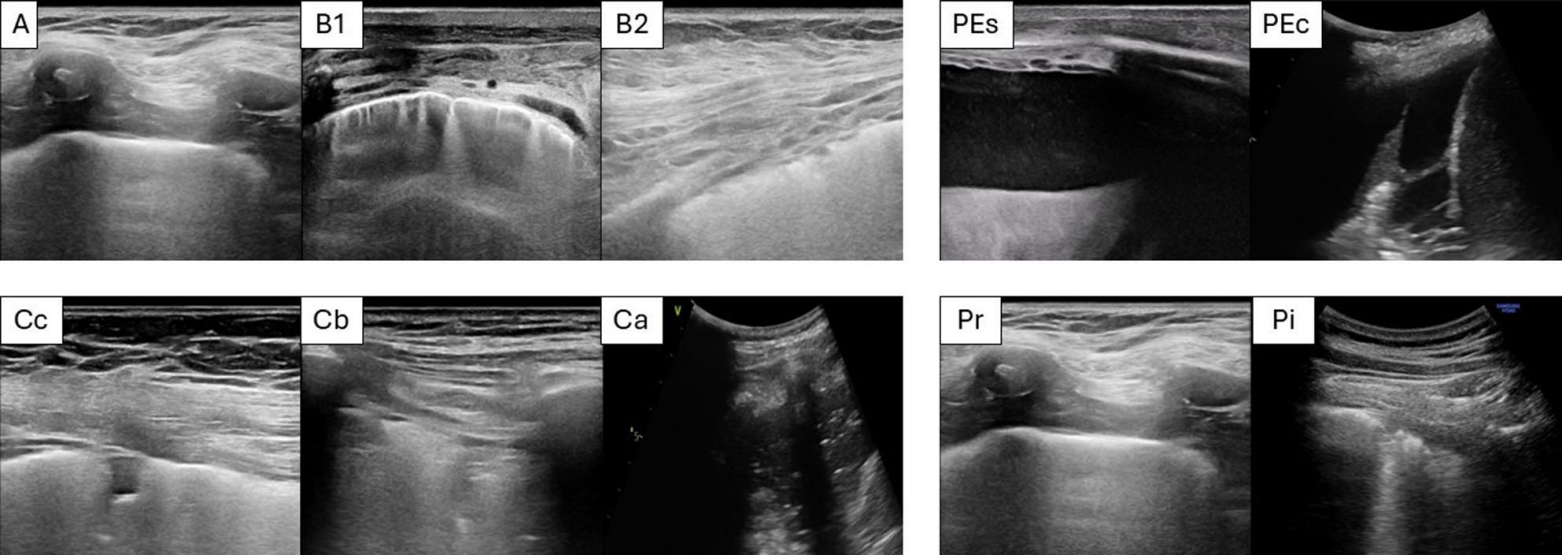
Lung ultrasound assessment. Left upper panels—from left to right: A pattern (A), at least more than 3 well-spaced B-lines visible in < 50% of the visualized pleura (B1), confluent B lines (B2). Right upper panels—from left to right: simple pleural effusion (PEs), complicated pleural effusion (PEc). Left lower panels—from left to right: small subpleural consolidation 0.5–1.0 cm in diameter (Cc), lung consolidation with dynamic bronchogram (Cb), lung fields with consolidation with no bronchogram or only static air or fluid bronchogram(s) (Ca). Right lower panels—from left to right: normal pleura (Pr), irregular pleura (Pi)
